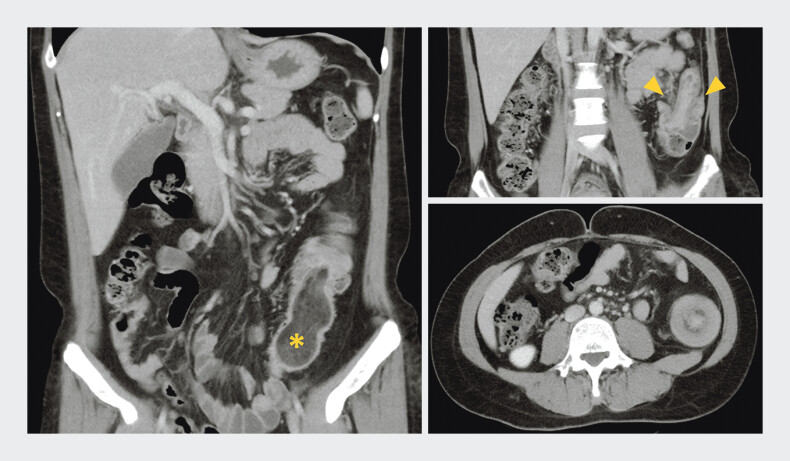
Abdominal computed tomography showing a hypodense submucosal mass (asterisk) in the descending and sigmoid colon with intussusception (arrowhead).
